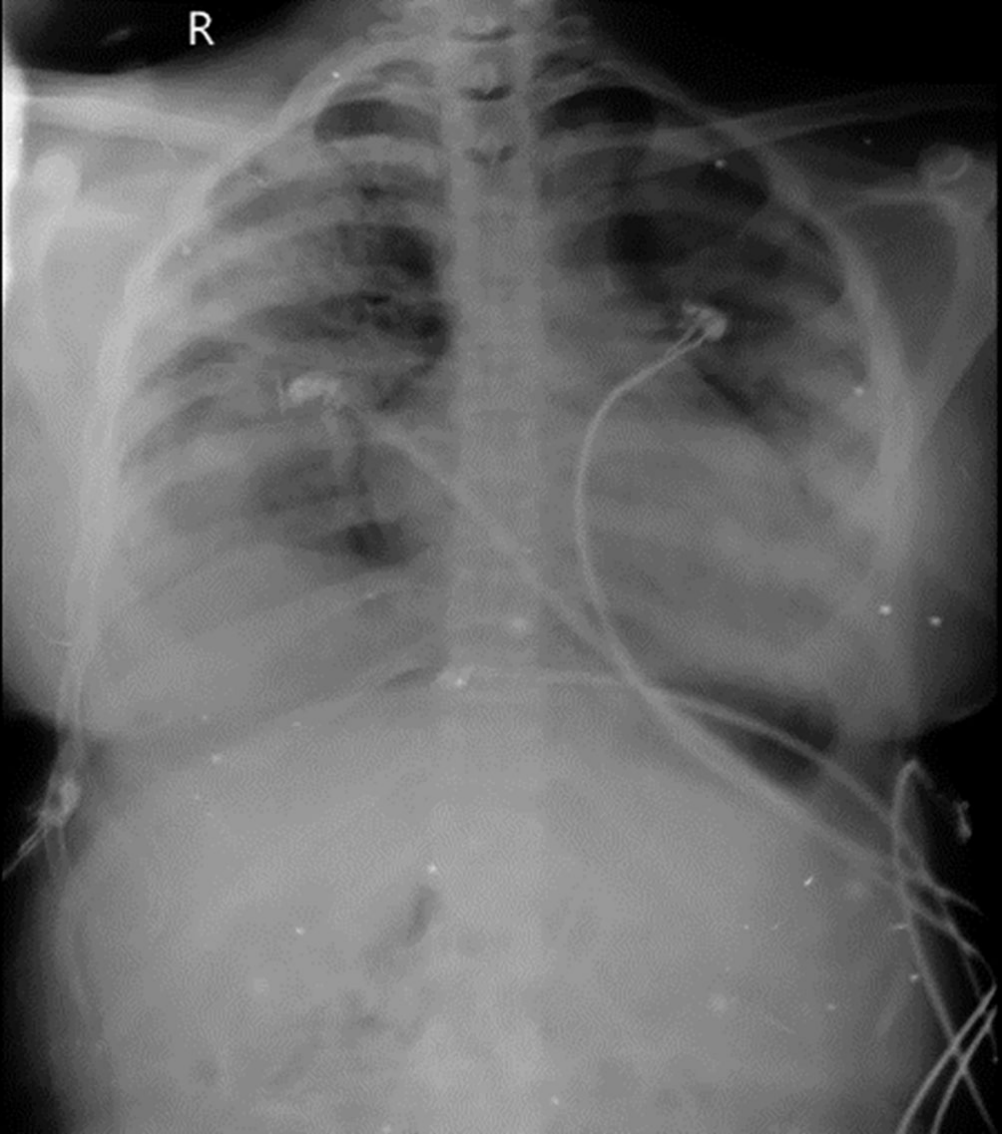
Chest radiograph PA view, day 5.
